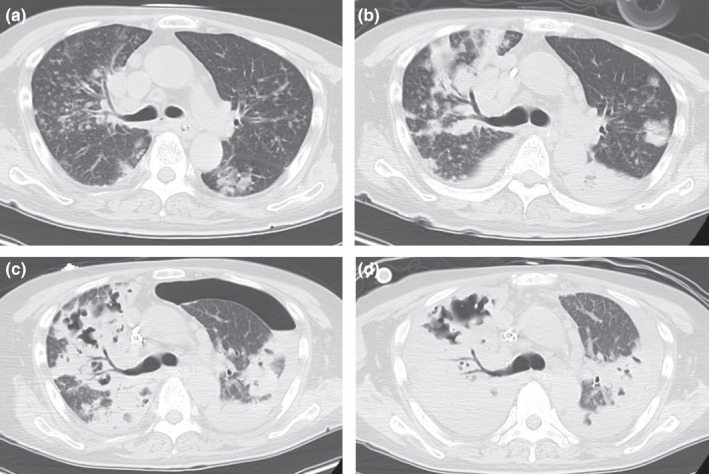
Computed tomography (CT) showing rapid progression of the necrotizing pneumonia caused by methicillin‐resistant *Staphylococcus aureus*, despite appropriate antibiotic therapy. (A) Plain CT showing bilateral multilobular lung nodules and bronchial wall thickening on Day 1. (B) Chest CT showing the expansion of bilateral consolidation on Day 3. (C) Chest CT showing necrotizing pneumonia with a left hydropneumothorax on Day 10. (D) Chest CT showing expansion of the cavity in the patient's left lung on Day 13
